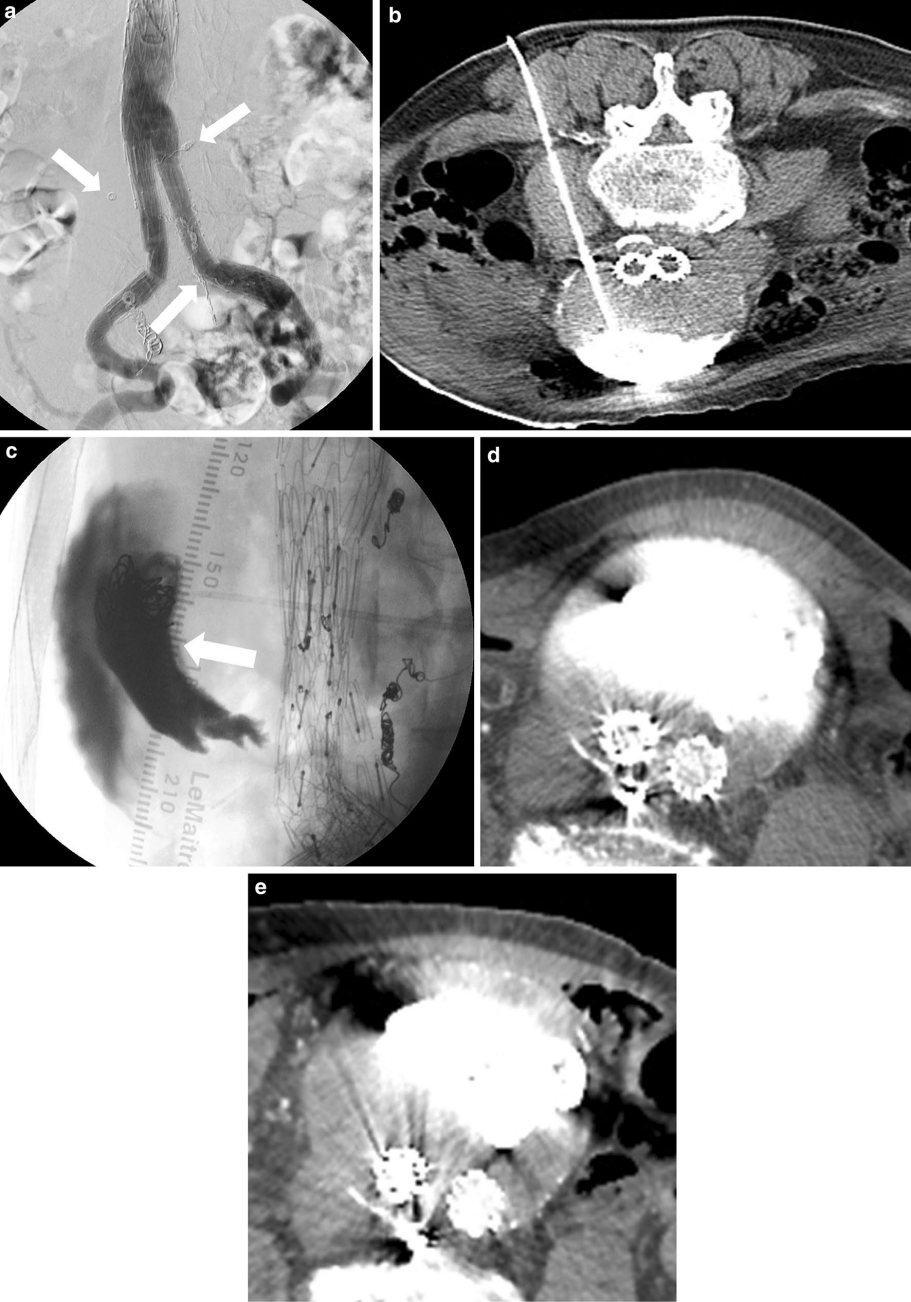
Images of an 87-year-old man with T2EL (case no. 6, Table 1). a Angiography in the prone position showed no endoleak before the third embolization. Lumbar artery and middle sacral artery were filled with coils (*arrow*) as a result of previous TAE. b Plain CT showed that the tip of the sheath that had been advanced after direct puncture under fluoroscopic guidance was correctly located. c Roentgenogram obtained after the third embolization showed NBCA and coils (*arrow*) in the endoleak sac. d Enhanced CT obtained 5 days after the third embolization showed NBCA and contrast media in the endoleak sac. Diameter of the aneurysm was 80 mm. e Enhanced CT obtained 6 months after the third embolization showed no endoleak; however, growth of the aneurysm sac was confirmed. Diameter of the aneurysm was 83 mm
